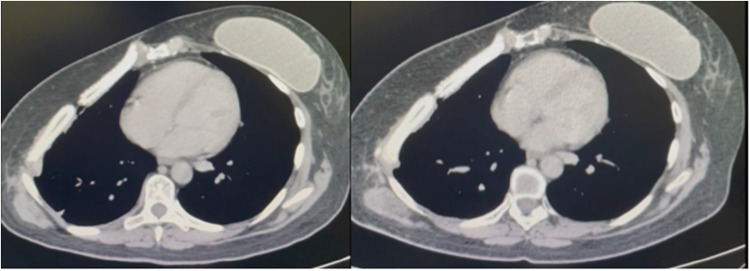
CT-Scan showing the reconstruction after three- and seven-months respectability.
